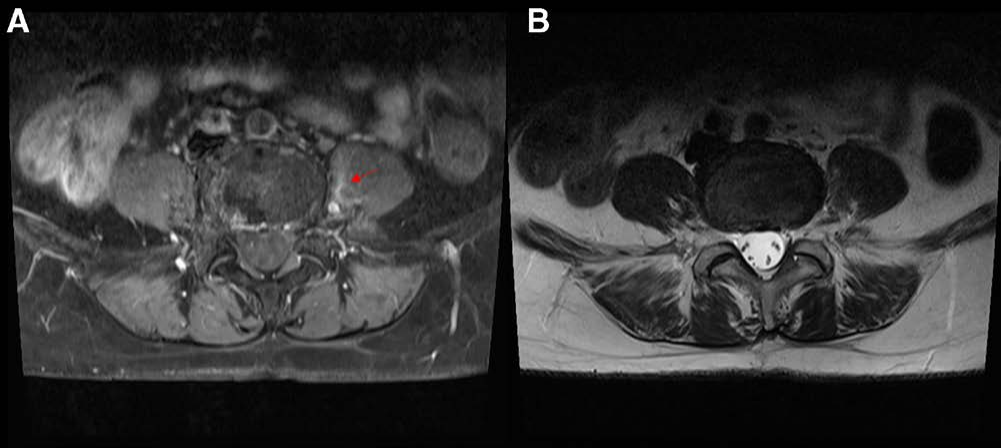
(A) T1-weighted gadolinium-enhanced axial MRI shows increased intensity in left psoas muscle (red arrow) (B) Conventional T2-weighted MRI shows no abnormal finding in left psoas muscle. MRI, magnetic resonance imaging. MRI = magnetic resonance imaging.
